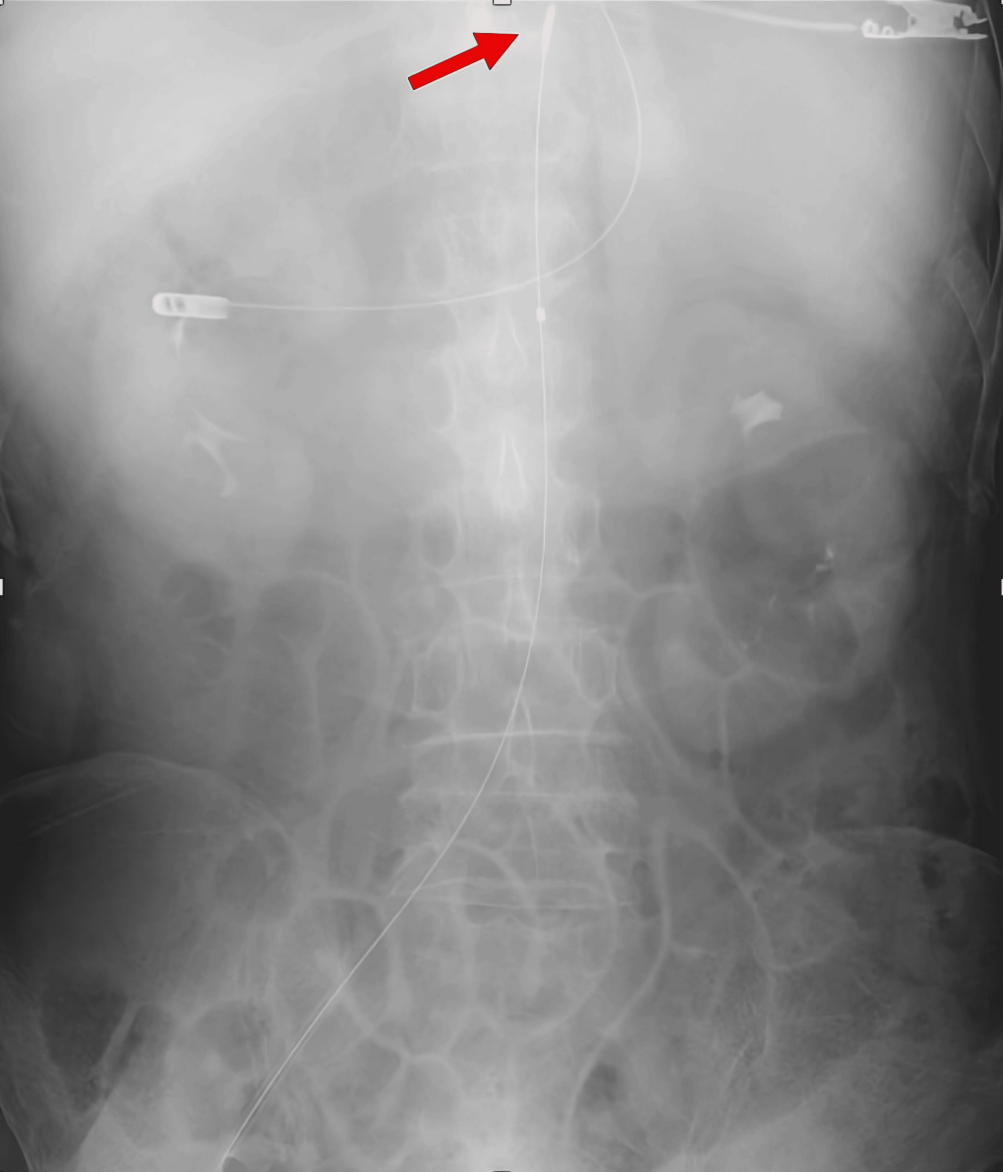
Chest X-ray demonstrating the REBOA catheter (Case 3) The red arrow indicates the tip of the REBOA catheter, which is appropriately positioned. REBOA: Resuscitative endovascular balloon occlusion of the aorta
